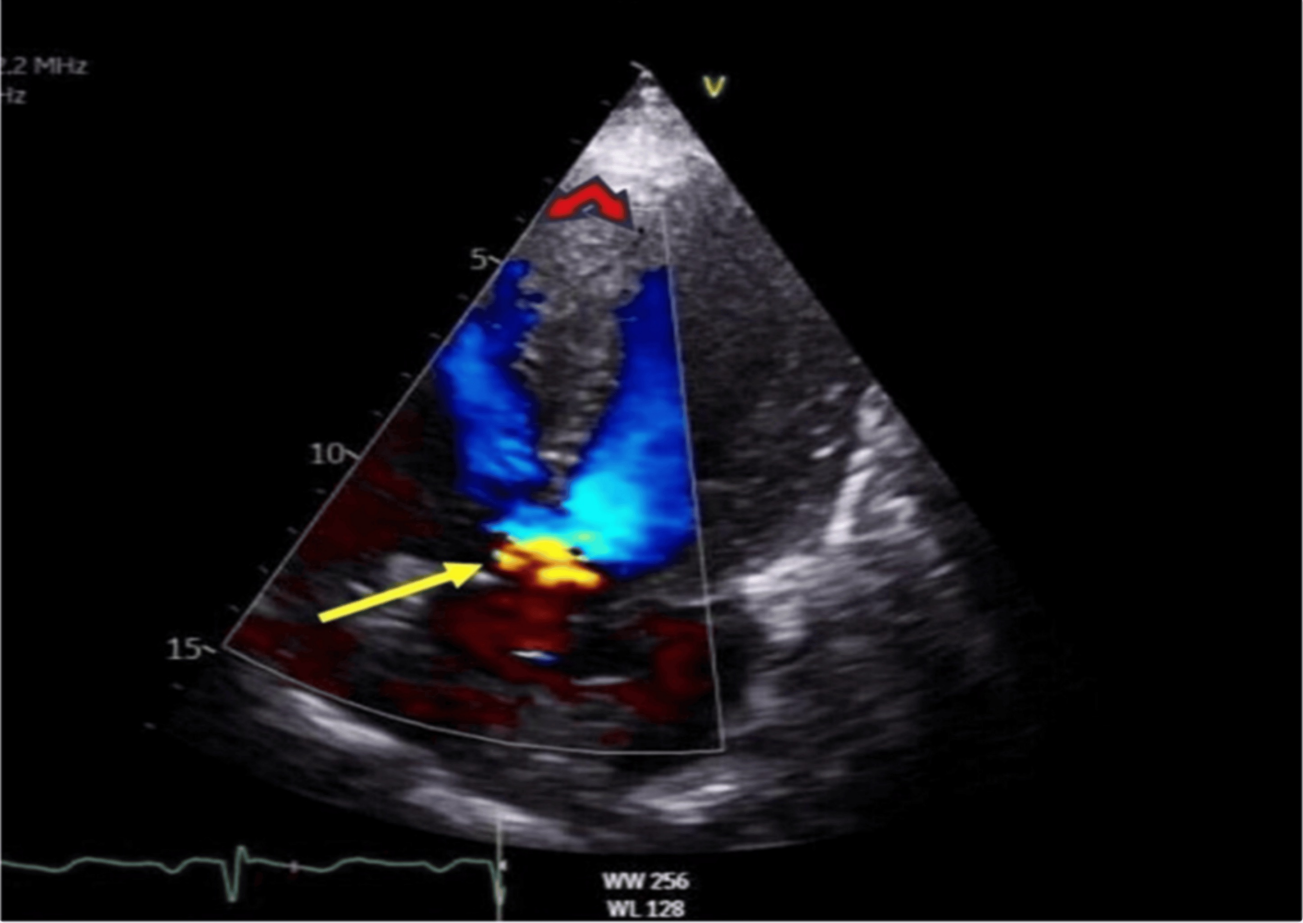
Yellow arrow, large VSD with bidirectional flow, a hallmark feature of Eisenmenger indicating significant right ventricular pressure. Red double arrow: backflow into both the left ventricle and right ventricle simultaneously. Doppler effect-apical four-chamber view VSD: ventricular septal defect; LV: left ventricle The left ventricle was normal in size and thickness. Additionally, the echocardiogram displayed an overriding aorta, shown in Figure 3. Echo revealed good systolic function and an LV ejection fraction of 55%
